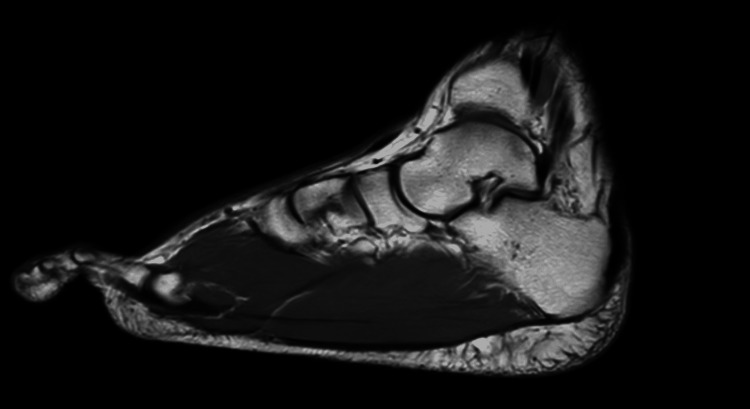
Preoperative MRI showing hypertrophy of multiple intrinsic foot muscles.
